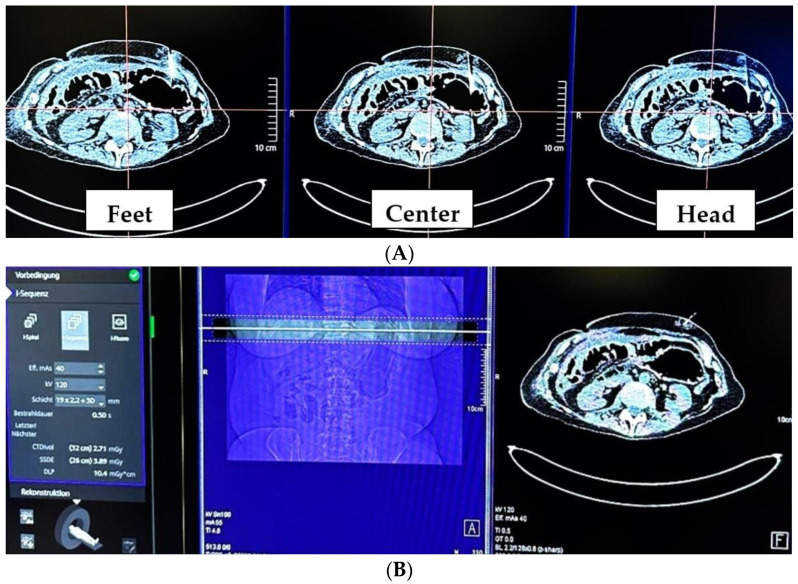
MS-CT biopsy mode (A) This screenshot was captured during an MS-CT biopsy mode-guided procedure, showing the image output used by the interventionalist to plan the next steps. When activated by the radiographer using the foot pedal, the CT scanner acquires and displays images of three adjacent sections. The upper image corresponds to the level of the gantry laser beam visible on the patient’s surface. The anchor needle runs from bottom to top. (B) Screenshot of the planning console. Patient positioning and scan area are visible. In addition, the acquired images can also be viewed one by one.
